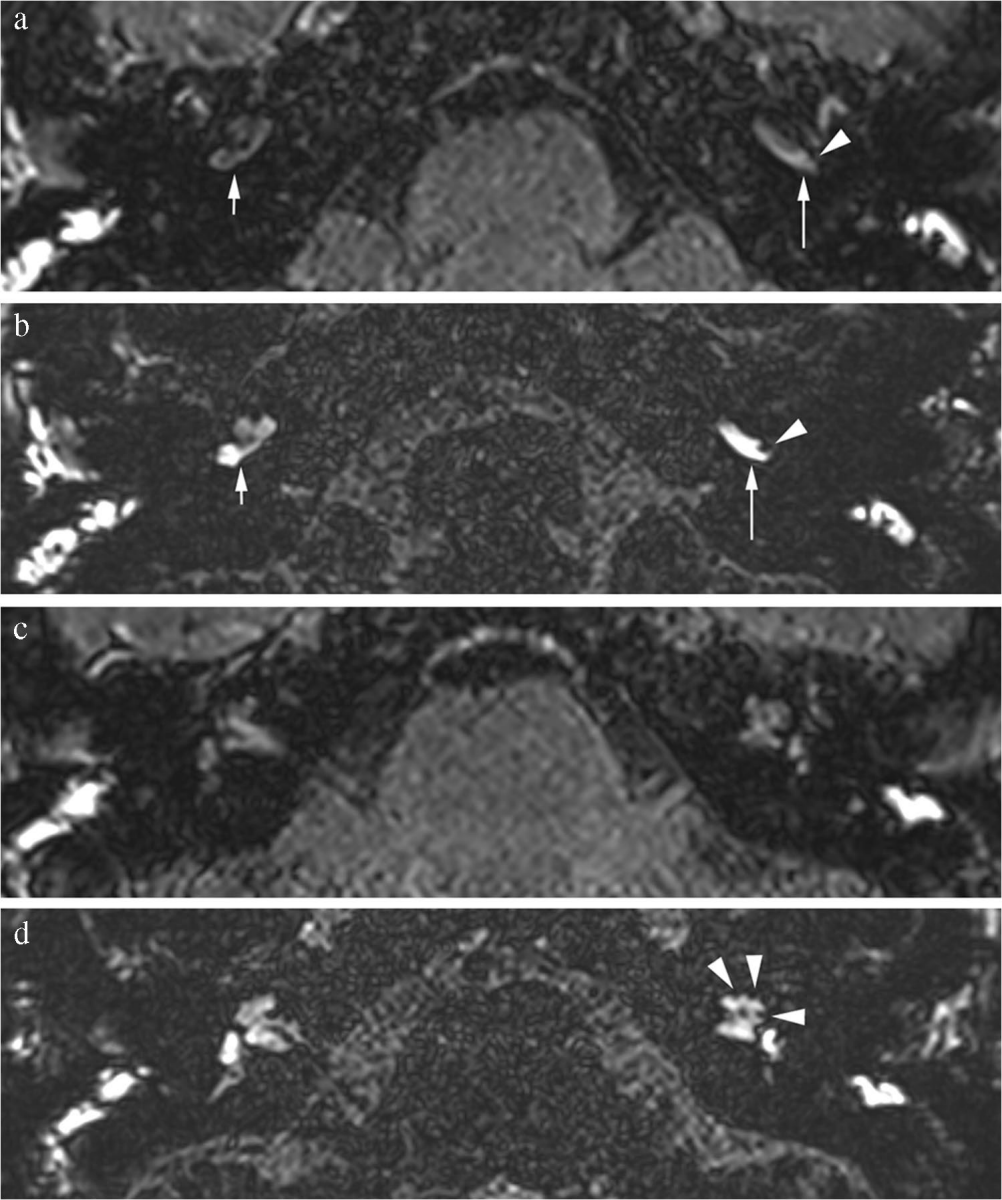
Forty-two-year-old female with a recent history of vertigo attacks associated with hearing loss and pressure sensation in the left ear. Audiometry reveals low-frequency sensory-neural hearing loss on the left side. Clinically, patient was classified as definite MD. Imaging performed 4 h after IV administration of a single dose of Gd. a Axial 3D TSE FLAIR image at the level of the basal turn of the cochlea. There is an asymmetrical perilymphatic enhancement, more pronounced on the left (large arrow) than on the right (small arrow). b Axial 3D SPACE FLAIR image at the level of the basal turn of the cochlea. There is an asymmetrical perilymphatic enhancement, more pronounced on the left (large arrow) than on the right (small arrow), which is also more clearly visible than on the 3D TSE FLAIR sequence. Note the slightly dilated endolymphatic space protruding as a small black cut-out (arrowhead) into the posterolateral aspect of the basal turn of the cochlea compatible with a grade 1 cochlear EH, which is not visible on the 3D TSE FLAIR sequence. Compare to Fig. 2a. c Axial 3D TSE FLAIR image at the level of the mid and apical turn of the cochlea shows no clear abnormalities. d Axial 3D SPACE FLAIR image at the level of the mid and apical turn of the cochlea showing the dilated endolymphatic space appearing as small black cut-outs (arrowheads) in the periphery of the mid and apical turn of the cochlea, compatible with a grade 1 cochlear EH. These abnormalities cannot be seen on the axial 3D TSE FLAIR sequence. Compare to Fig. 2c. A grade 1 vestibular hydrops in this patient was also seen (not shown)
